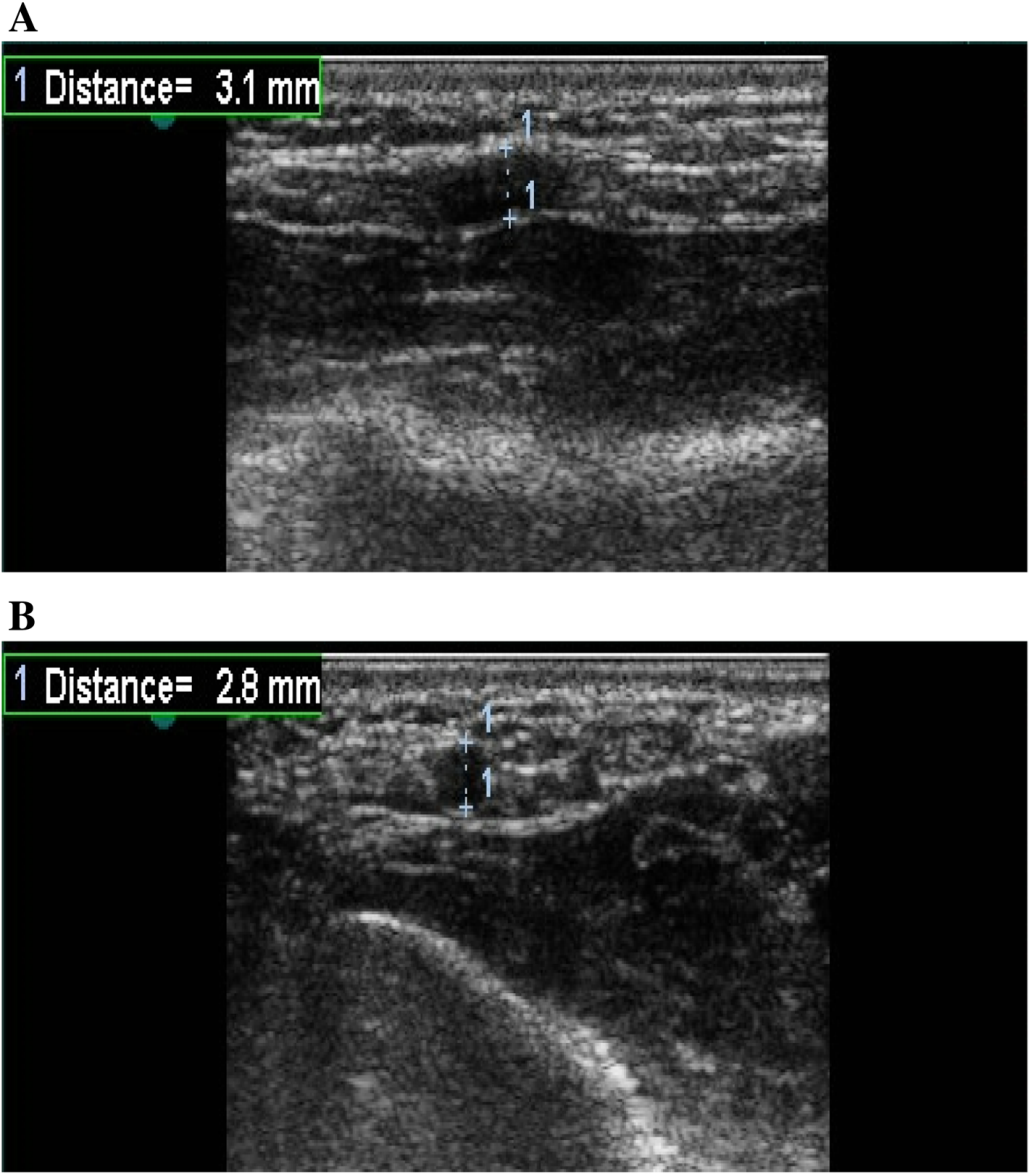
Ultrasonography of the RA using the B-mode. a Diameter of the RA in the horizontal section. b Diameter of the RA in the longitudinal section
